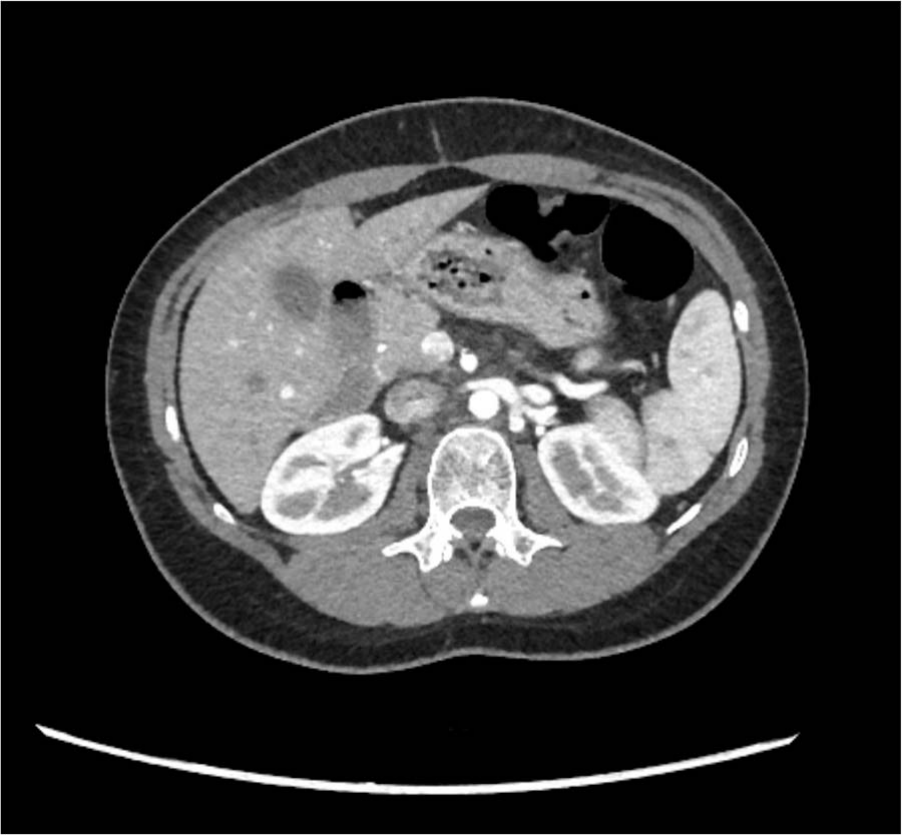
CT pancreas demonstrating absent body and tail of pancreas in axial view.
